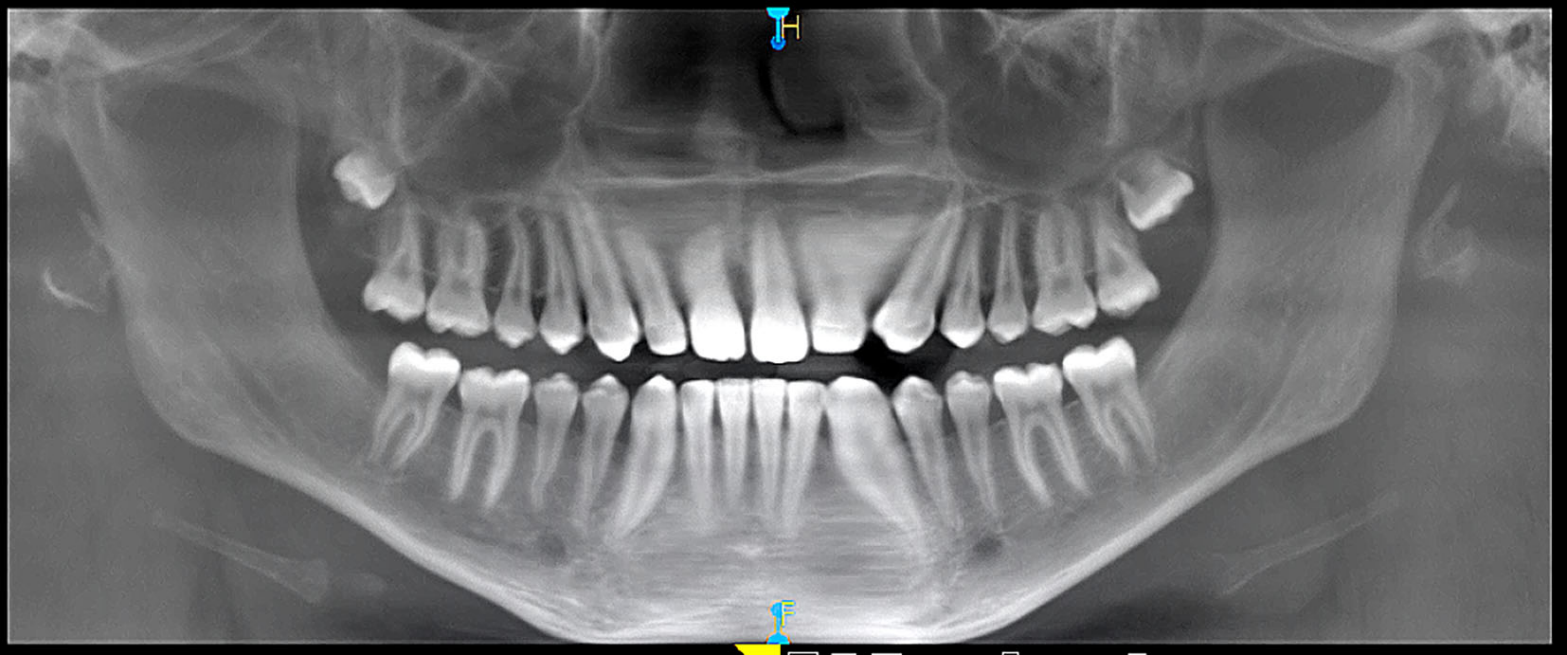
Panoramic radiograph (OPG) two years postoperatively.
